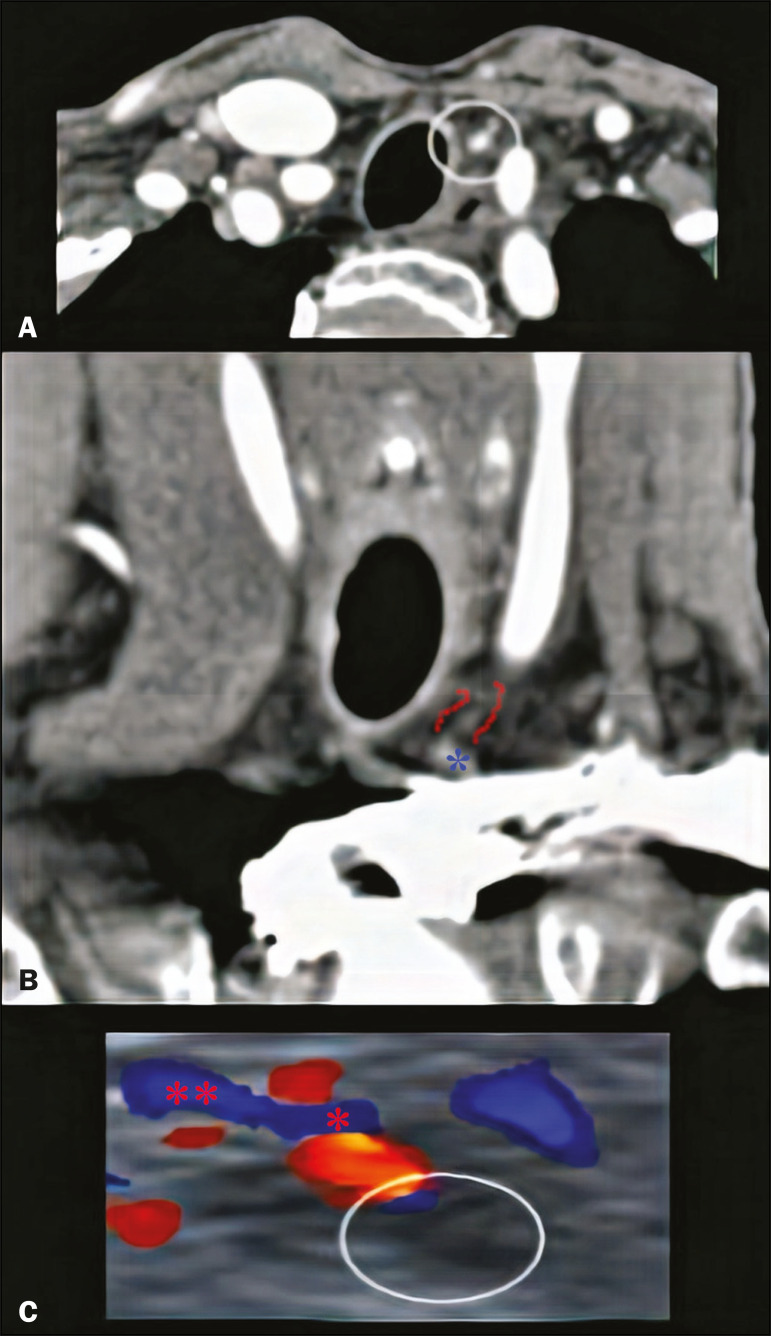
Parathyroid adenoma. A: Contrast-enhanced CT scan acquired in the arterial phase, showing a nodule with avid enhancement (circle) in the left thyroid bed. B: Contrast-enhanced coronal CT scan acquired in the arterial phase, showing a polar vessel (between the red lines) feeding a nodule with avid enhancement (blue asterisk). C: Correlation with ultrasound; color Doppler showing a hypoechoic area in the left thyroid bed (circle), as well as the polar feeding vessel (red asterisks).
